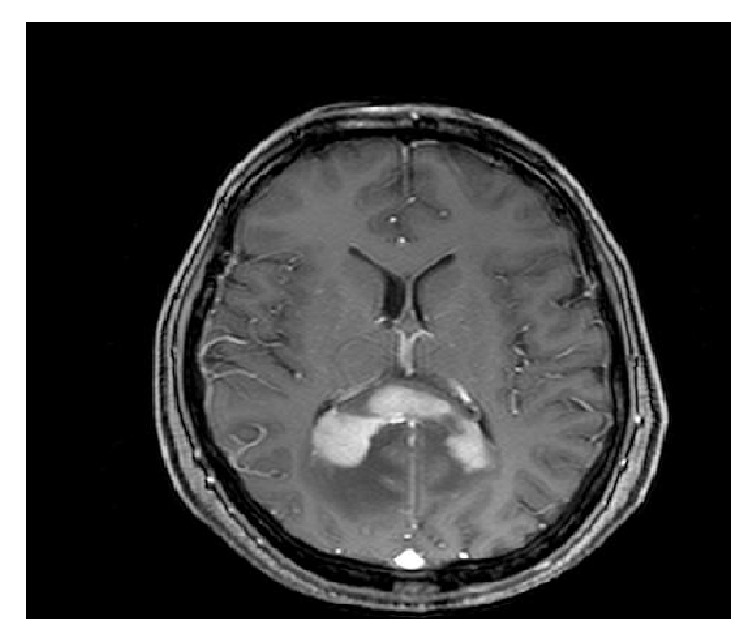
Contrast-enhanced axial T1-weighted MRI showing a PCNSL located in corpus callosum.
